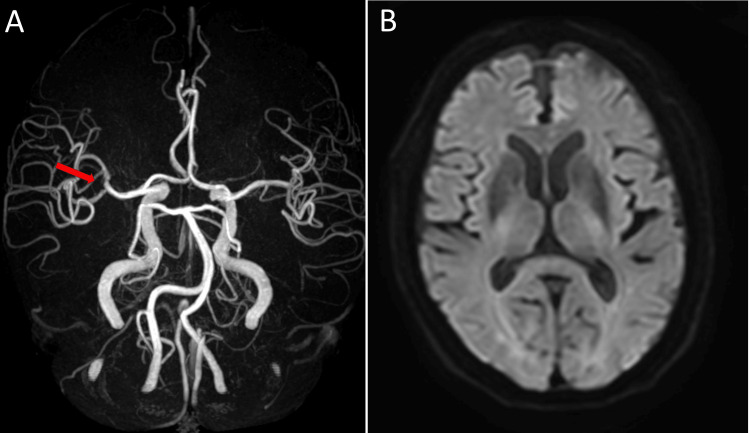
Post-interventional images. A: Magnetic resonance angiogram (MRA) indicates that the right middle cerebral artery (MCA) aneurysm has disappeared (red arrow). B: The diffusion-weighted imaging (DWI) showed no signs of hyperintensity in the cerebrum.
